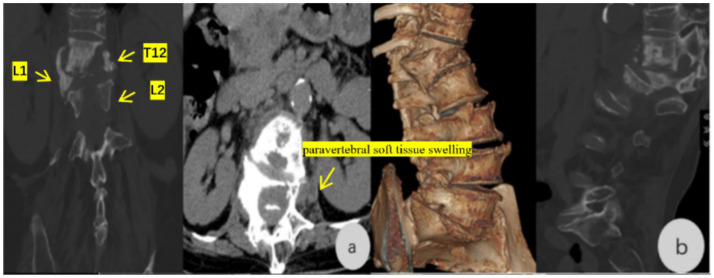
(a,b) Lumbar spine CT: Abnormal bone marrow signals (mixed lucency and sclerosis) in the T12–L2 vertebral bodies; paravertebral soft tissue swelling involving the ventral spinal canal.
